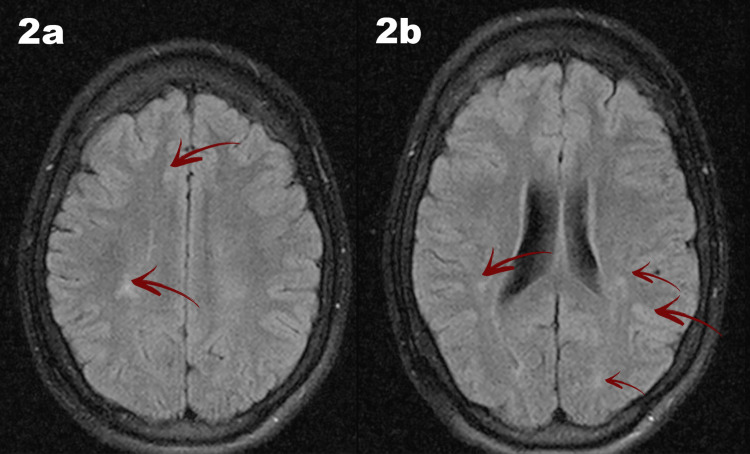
Brain magnetic resonance imaging (MRI) Brain MRI demonstrates mild asymmetry of the lateral ventricles, with the right ventricle appearing wider. Axial fluid-attenuated inversion recovery (FLAIR) images demonstrate hyperintense periventricular white matter lesions, more pronounced on the right side (Figures 2a–2b). Mild white matter volume reduction is present, consistent with gliotic changes likely related to a prior perinatal hypoxic–ischemic event. Notably, these findings occurred in the setting of an unusually early-onset and slowly progressive course, which is atypical for Lafora disease.
